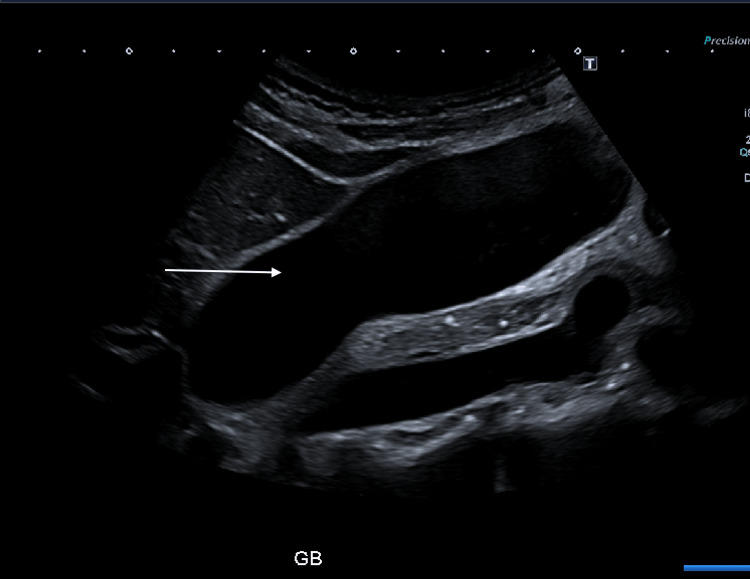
Longitudinal ultrasound image of the gallbladder showing a markedly distended, thin-walled gallbladder (arrow) without gallstones or pericholecystic fluid, consistent with reactive gallbladder distension secondary to acute pancreatitis
